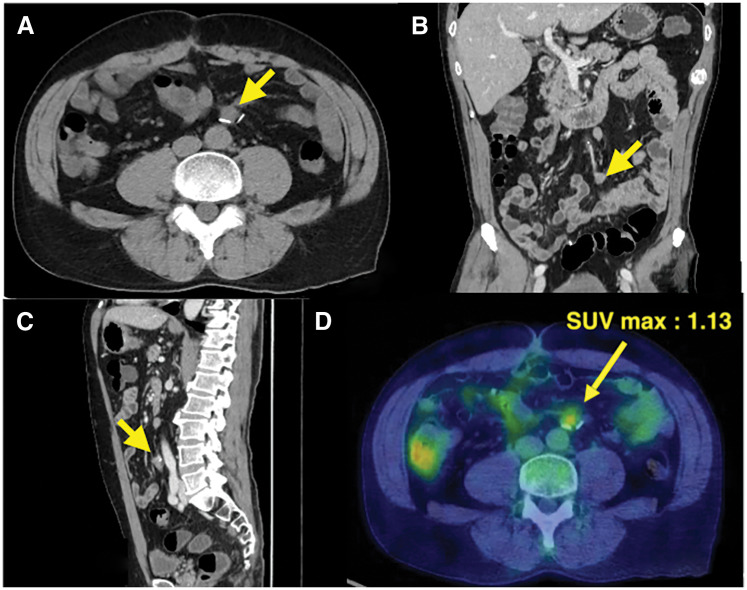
CT and FDG-PET images. (A–C) At 12 months postoperatively, CT images identified a 13-mm soft tissue lesion near the superior rectal artery stump (yellow arrow). (D) FDG-PET showed abnormal uptake at the stump of the superior rectal artery. A solitary lesion measuring 17 mm with a SUVmax of 1.13 was identified. FDG, ^18^F-fluorodeoxyglucose; SUVmax, maximum standardized uptake value
